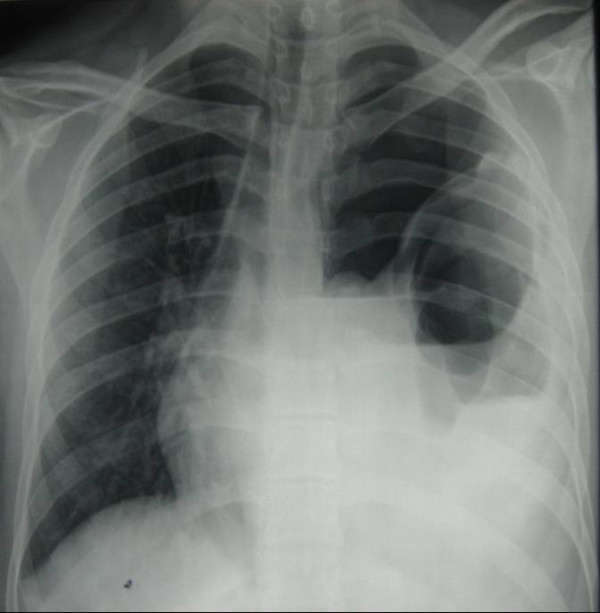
chest radiograph shows large colon in the left hemi-thorax with an air-fluid level. There is associated contralateral shift of the mediastinal contents.
